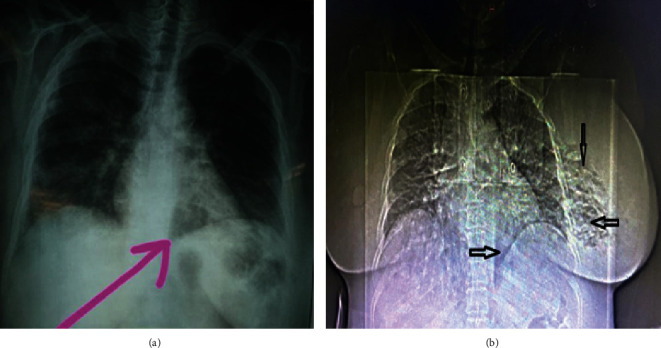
Chest X-ray posteroanterior view demonstrating suspicion of subcutaneous gas (see arrows).
